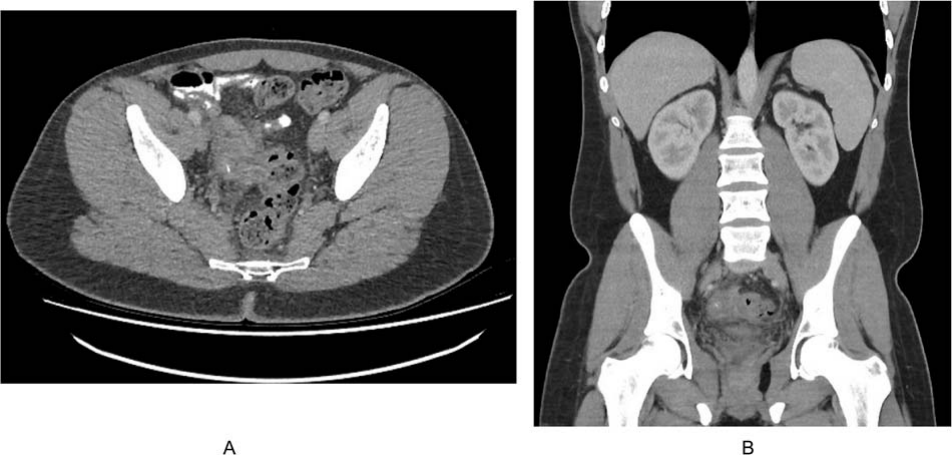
(A). Axial slice of a CT abdomen/pelvis in the portovenous phase demonstrating an inflammatory mass in the pelvis associated with the right pelvic side wall, with a calcific focus. (B). Coronal slice of the CT demonstrating the relation of the inflammatory mass within the pelvis.
